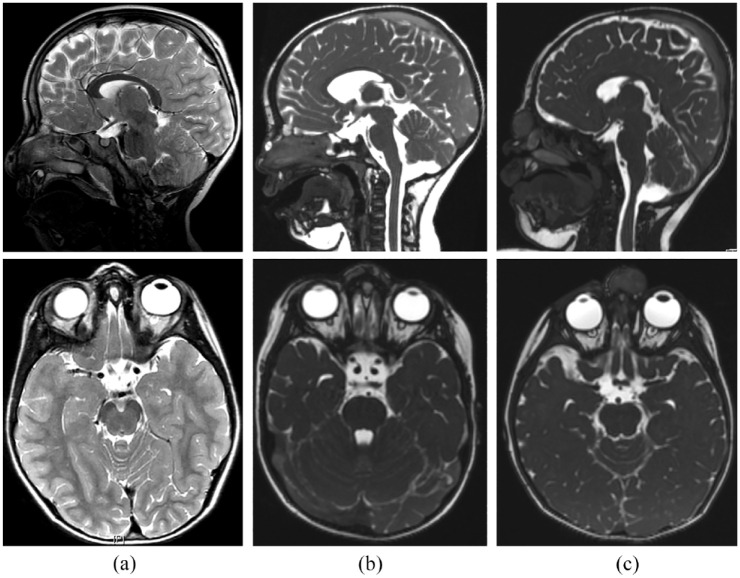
Preoperative imaging. T2-weighted sagittal and axial MRI scans of (a) mature teratoma with intracranial-extradural extension in patient P2, (b) 2 independent nasal dermoid sinus cysts in patient P7 with intraosseous and intracranial-intradural extensions, and (c) glial hamartoma with intraosseous extension in patient P16.
